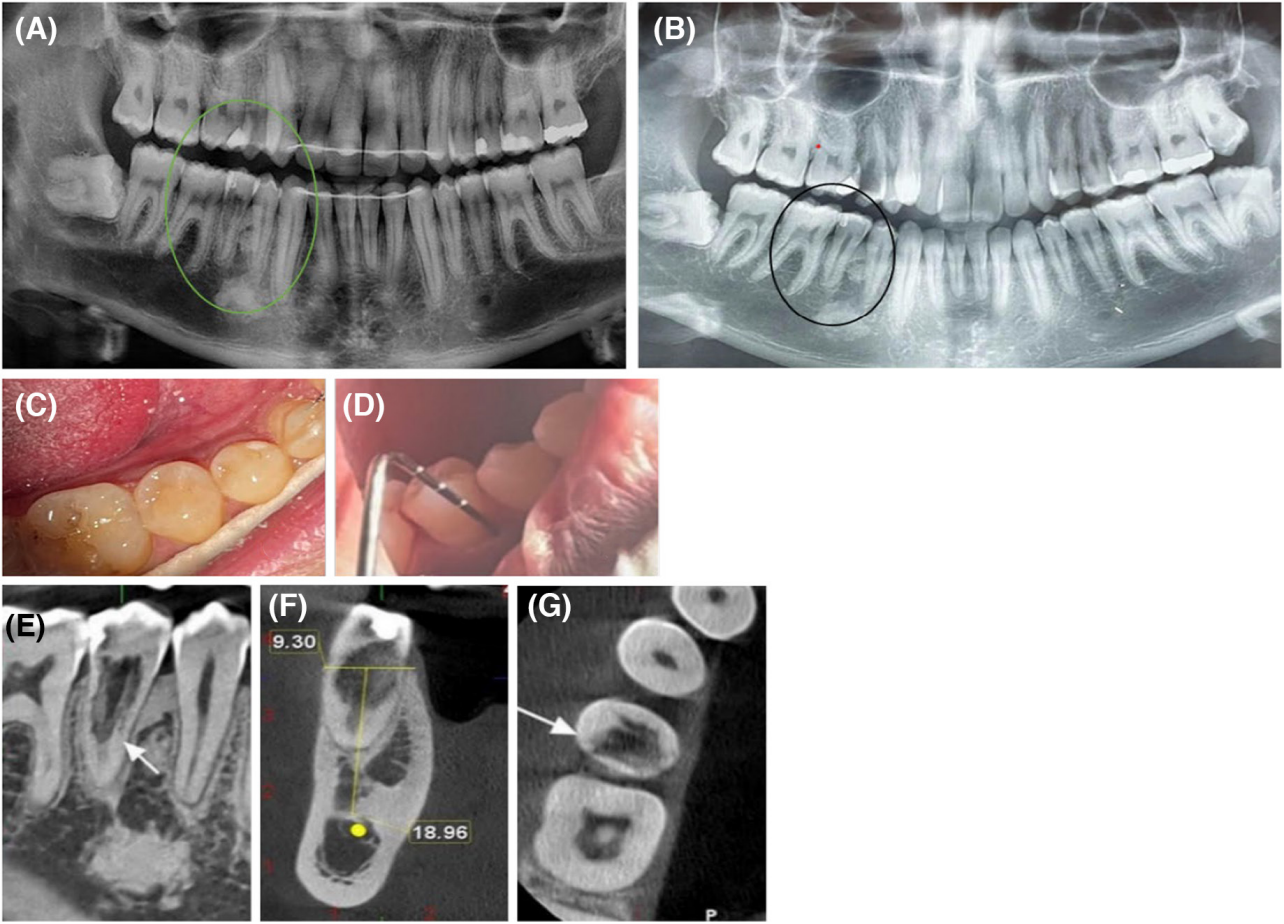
(A) panoramic radiograph after the end of orthodontic treatment. (B) Panoramic radiograph before orthodontic treatment, (C) intraoral examination, (D) probing depth, (E) periapical radiograph, (F) CBCT, coronal view, and (G) CBCT, axial view.
